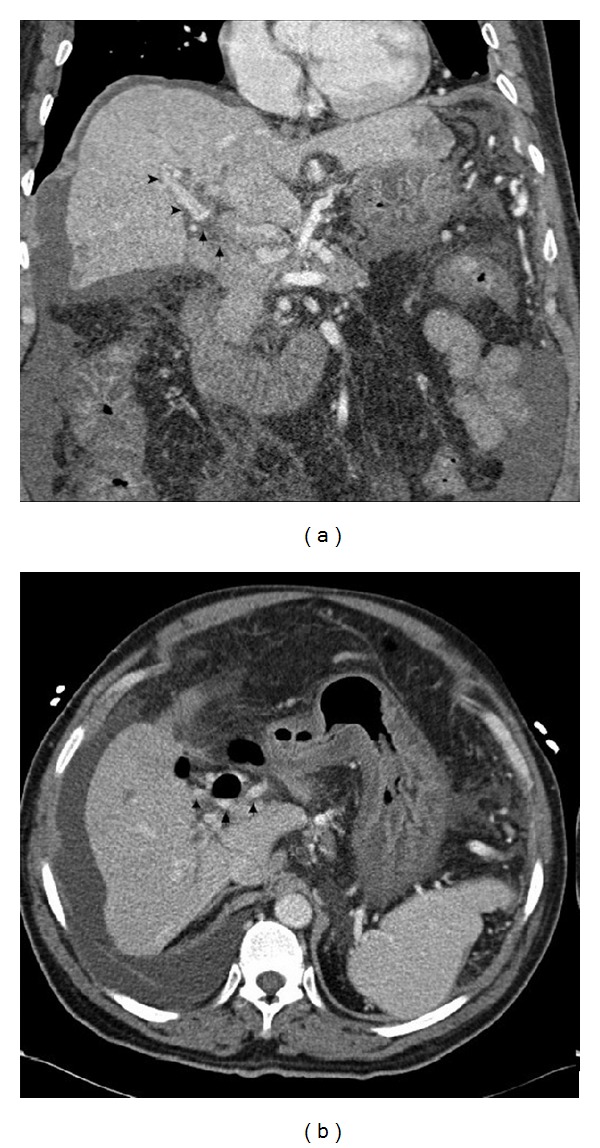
62-year-old man with portal vein thrombosis with cavernous transformation. Preprocedural coronal CT image demonstrates complete occlusion of the main portal vein (arrows), with reconstitution of intrahepatic branches (arrowheads) (a). Preprocedural axial CT image better demonstrates the multiple cavernous collaterals at the level of the hepatic hilum (arrows) (b).
